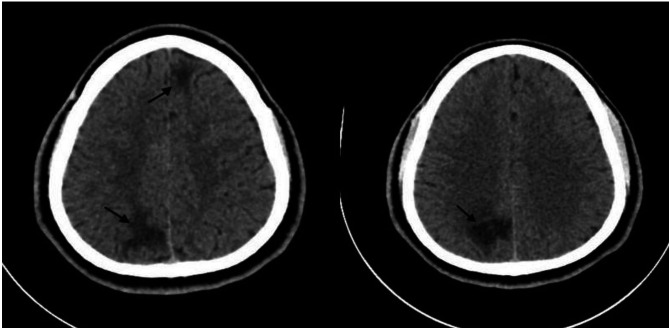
CT head showed two areas of hypodensity (black arrows) lesions representing vasogenic oedema involving the subcortical white matter of the left frontal and right posterior parasagittal location
